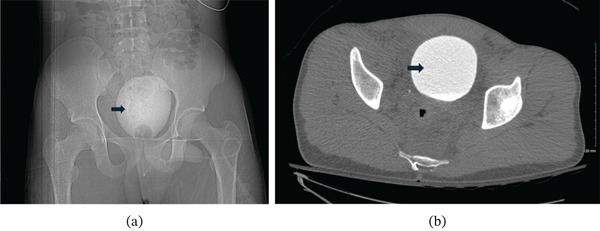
(a) CT scout view demonstrates contrast filled urinary bladder with its smooth and regular contour without extraluminal contrast material suggesting intact urinary bladder wall. (b) Axial pelvis image of retrograde CT urography suggests smooth contour of urinary bladder without evidence of bladder rupture. Arrows represent contrast filled urinary bladder.
